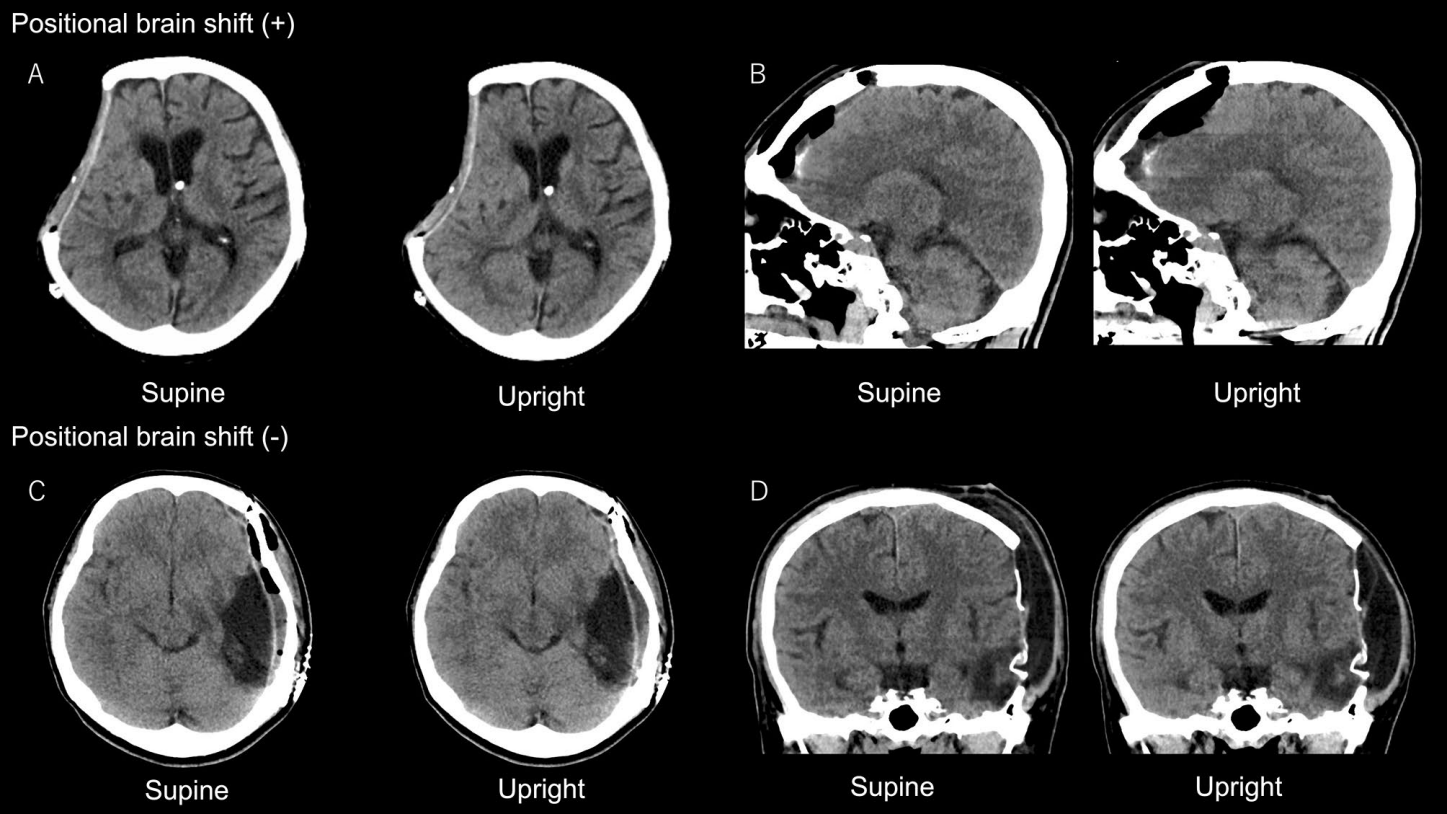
Examples of cases with (A, B) and without (C, D) positional brain shift (PBS). (A) A 70-year-old woman with ventriculoperitoneal shunt, who underwent craniectomy because of surgical site infection. Computed tomography (CT) scan images obtained on postoperative day (POD) 7 showed a remarkable PBS. (B) A 53-year-old man who underwent frontal craniotomy for clipping of a distal anterior cerebral artery aneurysm. In total, 14.9 mL of residual air, which moved upward according to positional change, thereby compressing the frontal lobe, was evident on CT scan images on POD 7. (C) A 41-year-old woman who underwent resection of left temporal glioma. CT scan images on POD 7 did not show PBS around the resection cavity. (D) A 55-year-old man who underwent craniectomy for traumatic brain injury. CT scan images on POD 17 did not show PBS. However, subcutaneous fluid collection indicated a positional shift.
